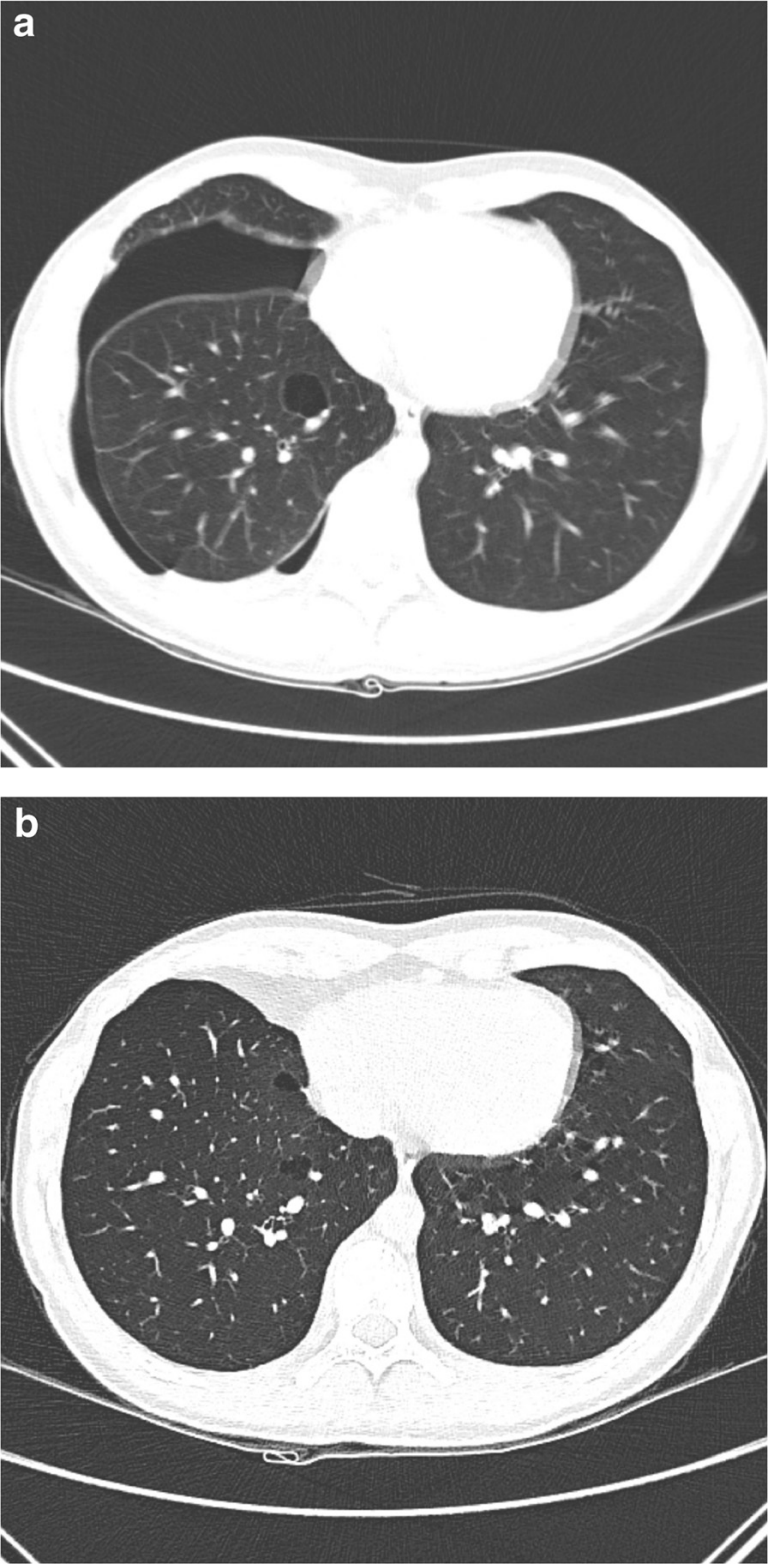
Thoracic CT of case 1. a. CT shows a pneumothorax with a intraparenchymal located cyst with small septa. b. Shows multiple cysts in the lower lobes (under the carina), in the same patient after treatment of the pneumothorax.
